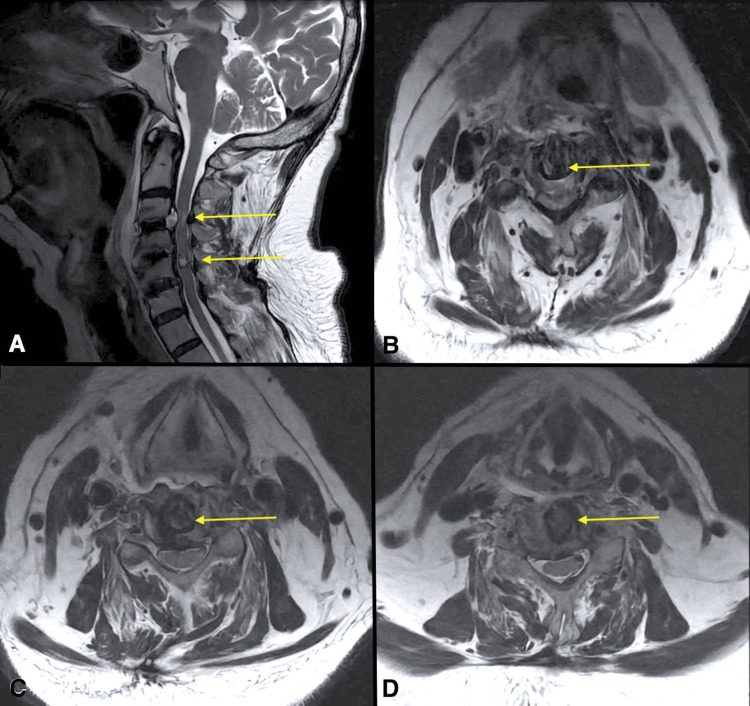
Postoperative (ACDF) T2-weighted MRI with sagittal and axial reconstructions Sagittal T2-weighted MRI showing (A) postoperative changes with intersomatic spacers C3-C4, C4-C5, and C5-C6, (B) axial postoperative changes of discectomy in C3-C4, and (C) C4-C5 and (D) C5-C6 intersomatic spaces. Central intramedullary cavernoma is observed (yellow arrows point to the lesions described). ACDF: anterior cervical discectomy with cage-augmented fusion; MRI: magnetic resonance imaging
